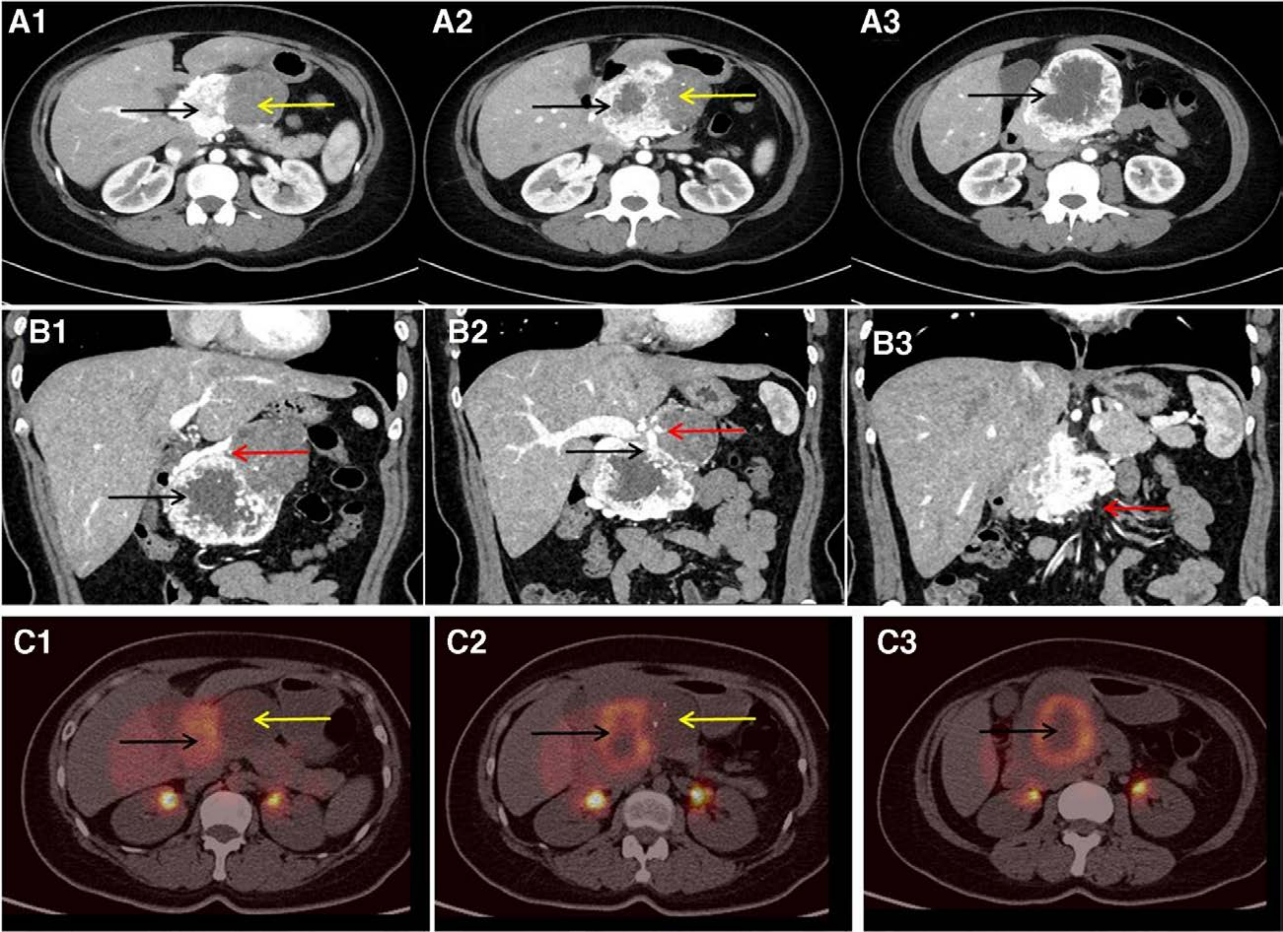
Abdominal enhanced computed tomography (CT) and PET-CT images. A1-A3: Axial enhanced CT images showed the enhanced mass (black arrow) with a nonenhanced cyst (yellow arrow) involving the head and body pancreas. B1-B3: Coronal reconstruction enhanced CT images displayed the mass (black arrow) invading its surrounding important blood vessels (red arrow); C1-C3: Axial PET-CT images revealed the unevenly fluorodeoxyglucose (FDG) uptake (black arrow) mass with nonuptake portion (yellow arrow) in lesser omental sac.
